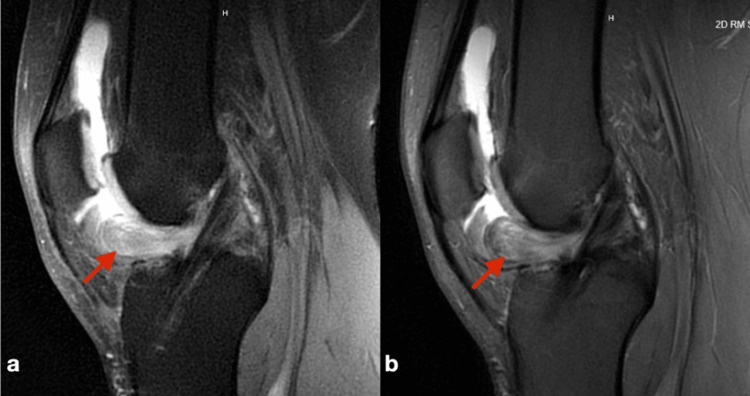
MRI images revealing the cyclops lesion a) T1 magnetic resonance imaging sagittal view of the left knee demonstrating the cyclops lesion (red arrow) anterior to the anterior cruciate ligament reconstruction. b) T2 magnetic resonance imagining of the same sagittal view with cyclops lesion (red arrow).
